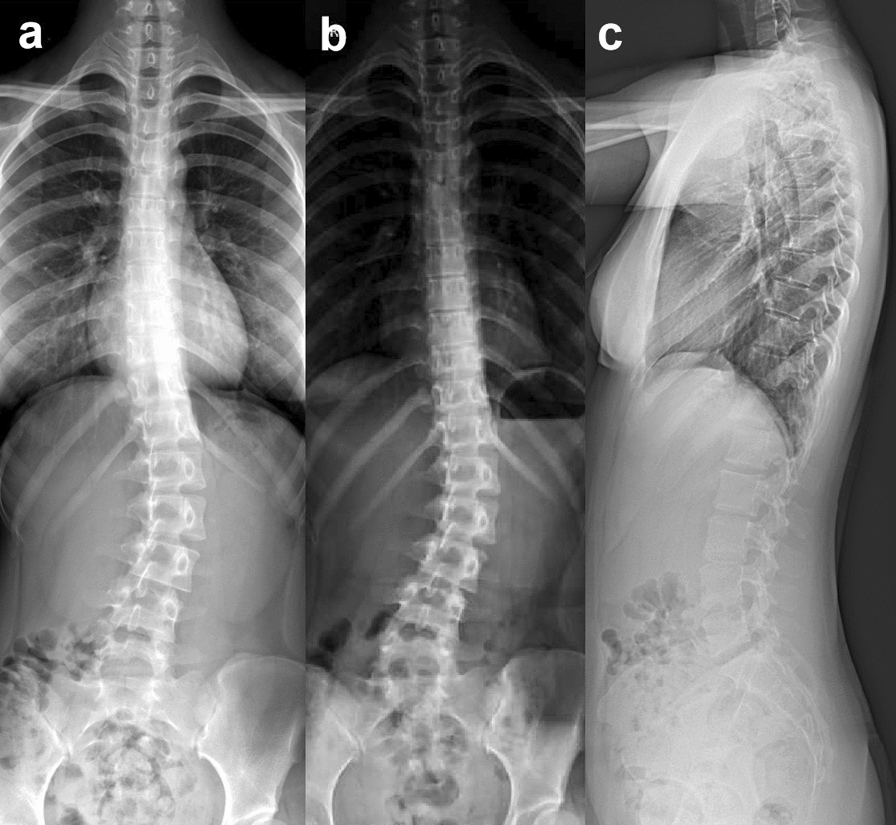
X-ray of the entire spine of a 17-year-old female showed Lenke 5C idiopathic scoliosis; a 1 year pre-admission; b, c on admission
